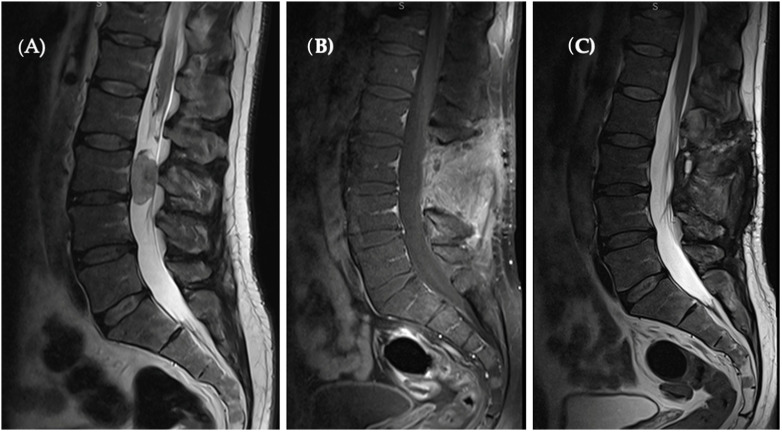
(A) Pre-op T2 sagittal MRI revealed a hyperintense oval-shaped lesion located at the L3 level occupying spinal canal. (B) Sagittal T1 with contrast enhanced as well as T2 sagittal. (C) Sections present a complete tumor removal and no sign of recurrence.
